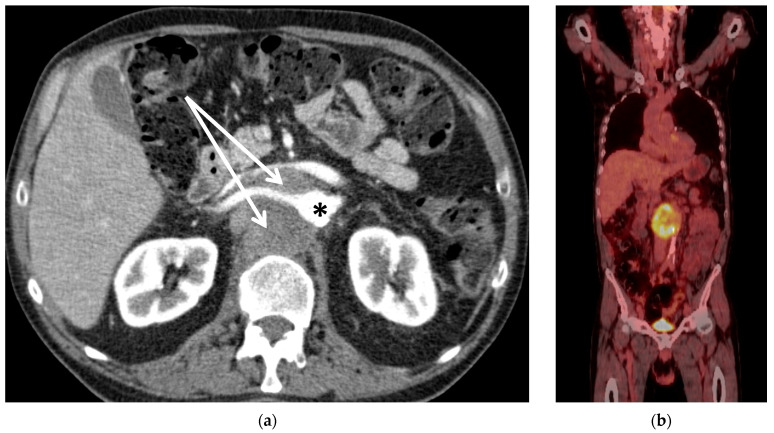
(a) CT scan of Patient #1 with retroperitoneal metastasis of unknown primary (arrows) encasing the aorta (*) and right renal artery. The left renal vein is compressed by the tumour mass. (b) Corresponding PET-CT scan with FDG-enhancement (yellow) at the site of the tumour and with the absence of further metastasis.
